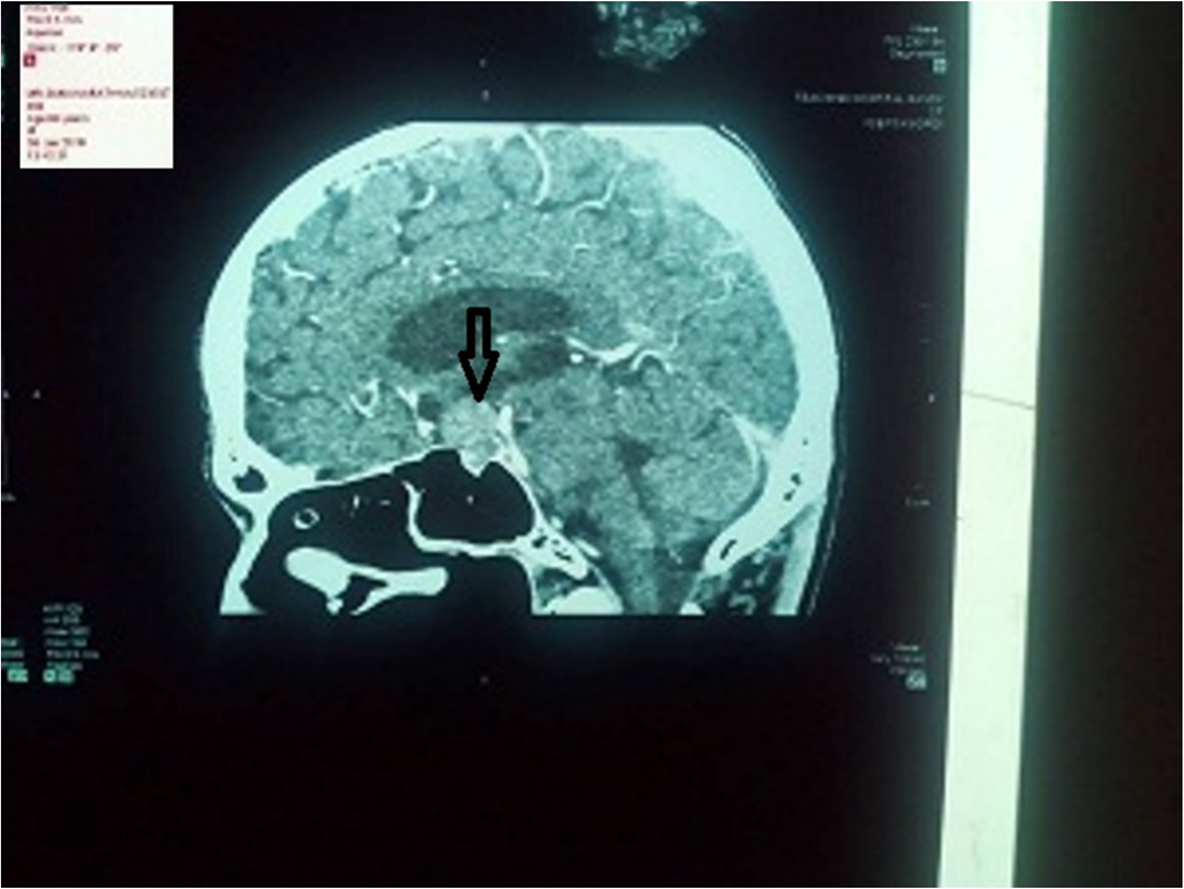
Sagittal section of CT brain showing suprasellar tumor suggestive of pituitary macroadenoma (arrow)
